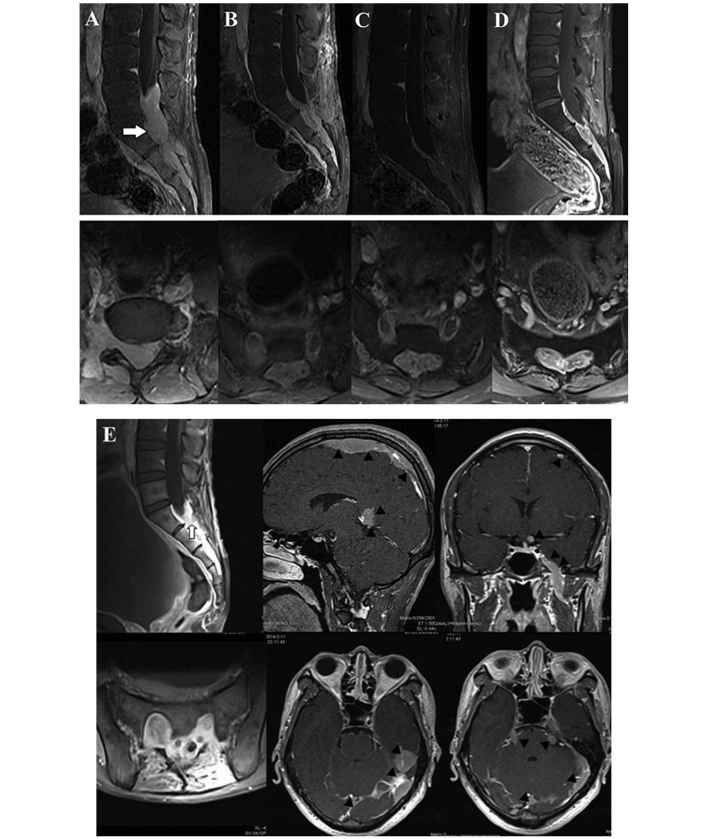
Sagittal and axial T2-weighted magnetic resonance imaging scans demonstrating the progression of the disease, including the treatment phase and the development of metastasis. (A) Sacral spinal mass at presentation extending from L5 to S2 associated with the destruction of adjacent soft tissues and bone strcutures (white arrow). (B) Post-surgery, and at the time of the first course of chemotherapy. (C) Following the second course of chemotherapy. (D) Ten months after the second course of chemotherapy. (E) Seventeen months after the second course of chemotherapy, showing metastasis to different sites of the brain (black arrowheads).
